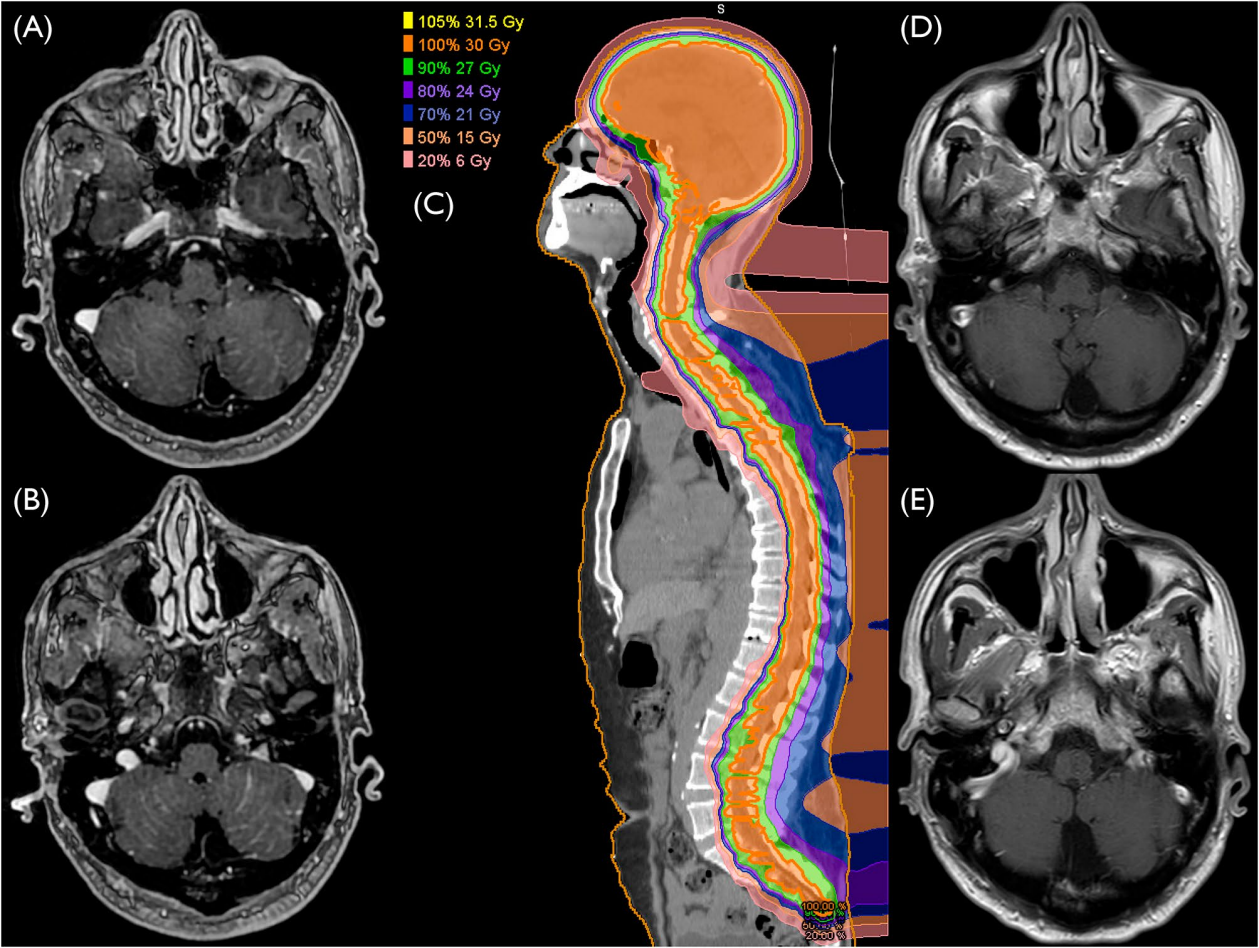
Case example. (A, B) 58-year-old male, KPS of 90%, with the diagnosis of extensive-stage small cell lung cancer and widespread cerebellar leptomeningeal disease on contrast-enhanced magnetic resonance imaging (MRI). He received chemotherapy with carboplatin and etoposide as well as immunotherapy with atezolizumab before and after proton craniospinal irradiation (pCSI) (C) pCSI treatment plan, 30 Gy (RBE) total dose, 10 fractions. (D, E) Three months after pCSI, contrast-enhanced MRI demonstrated complete resolution of LMD
